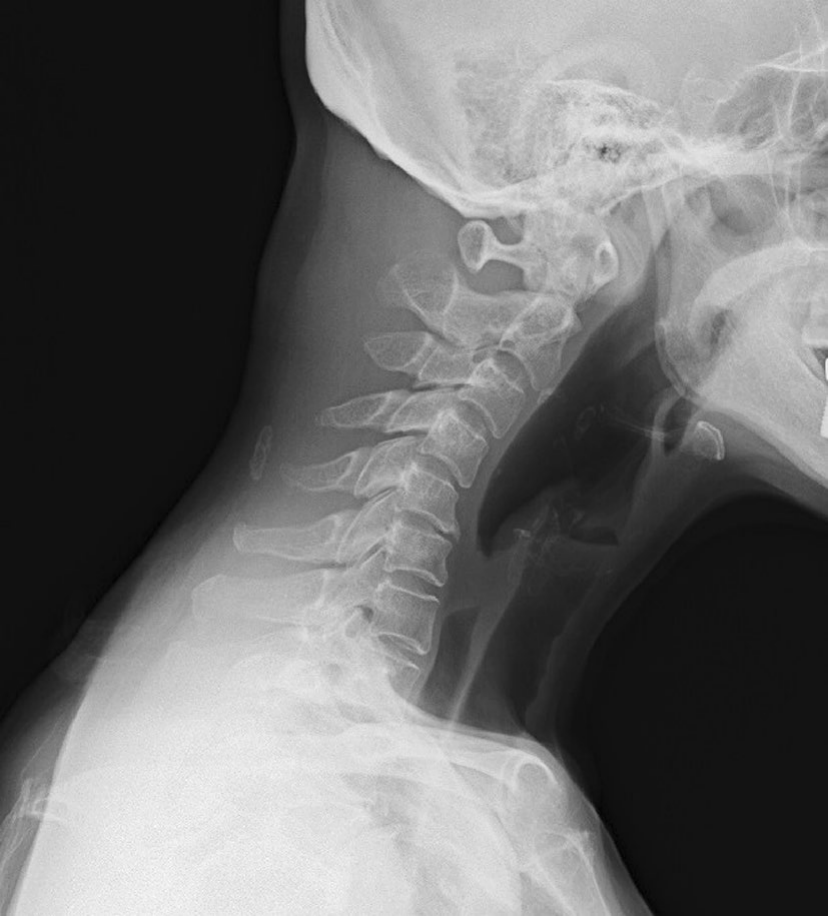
Lateral radiograph of cervical spine in a neutral position. Plain radiography shows severe cervical kyphosis at the patient’s first visit to our department
